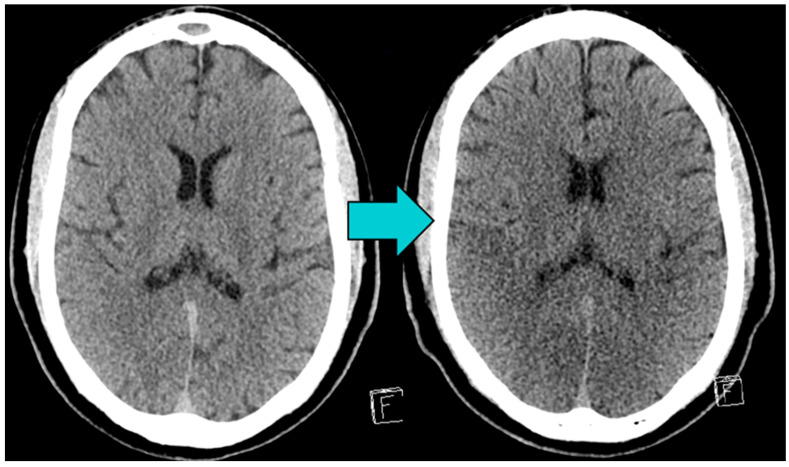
Images from a patient who suffered cardiac arrest (CA) and return of spontaneous circulation (ROSC). The initial unenhanced brain CT, taken < 6 h after ROSC (left image), shows no acute pathologies and no early signs of hypoxic-ischemic brain injury (HIBI). The follow-up brain CT (right image), taken 3 days after ROSC, shows global brain edema with diminished grey-matter white-matter differentiation and narrowed liquor rooms as signs of HIBI.
